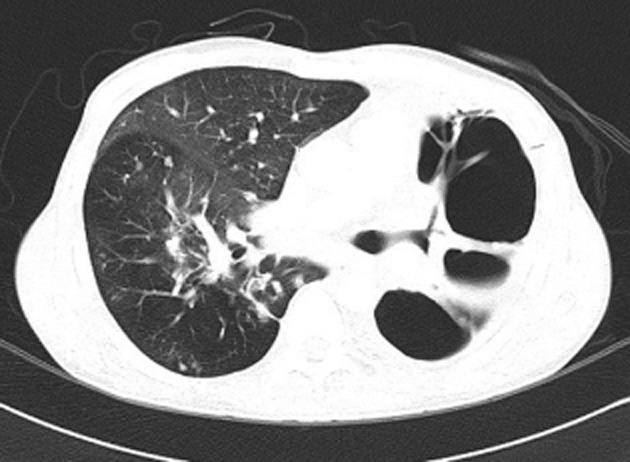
Computed tomography scan of the chest showed extensive lung destruction with multiple large thick-walled pulmonary cavities and severe fibrosis of the residual left lung. Diffuse fibro-nodular opacities were present in the right lung.
